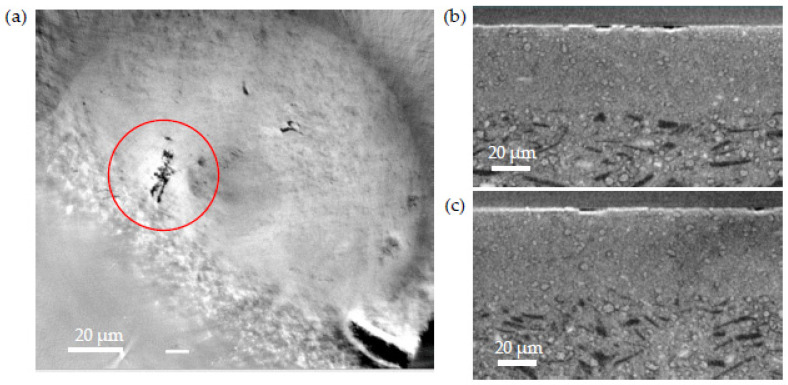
X-ray tomography images of a defect in the electrolyte of the reduced sample: (a) image from the top (*Y*-axis) of the defect in the electrolyte; (b) cross-section where the defect is along the *X*-axis; (c) cross-section where the defect is along the *Z*-axis.
